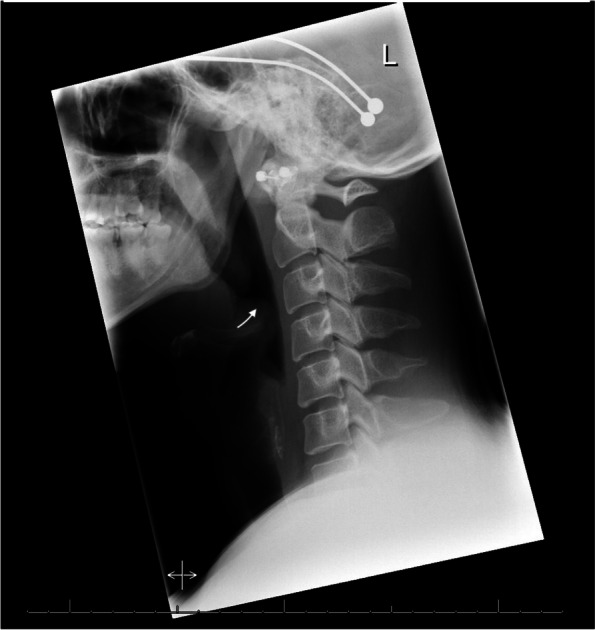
Lateral neck soft tissue radiograph: poorly defined and thickened epiglottis. The arrow points to the thumb sign which is commonly associated with acute epiglottitis
